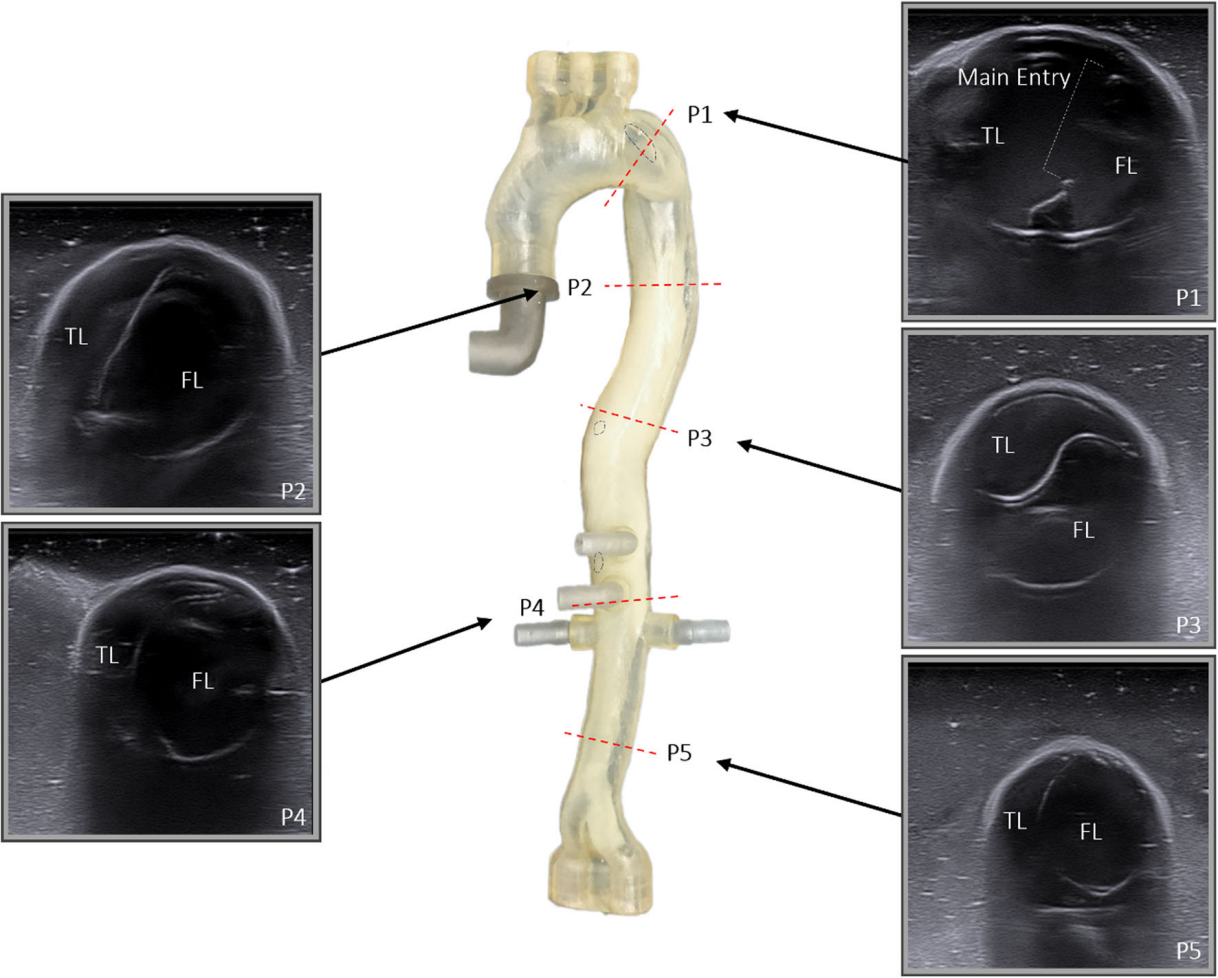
Ultrasound axial views with true lumen and (TL) false lumen (FL) divided by dissection flap
